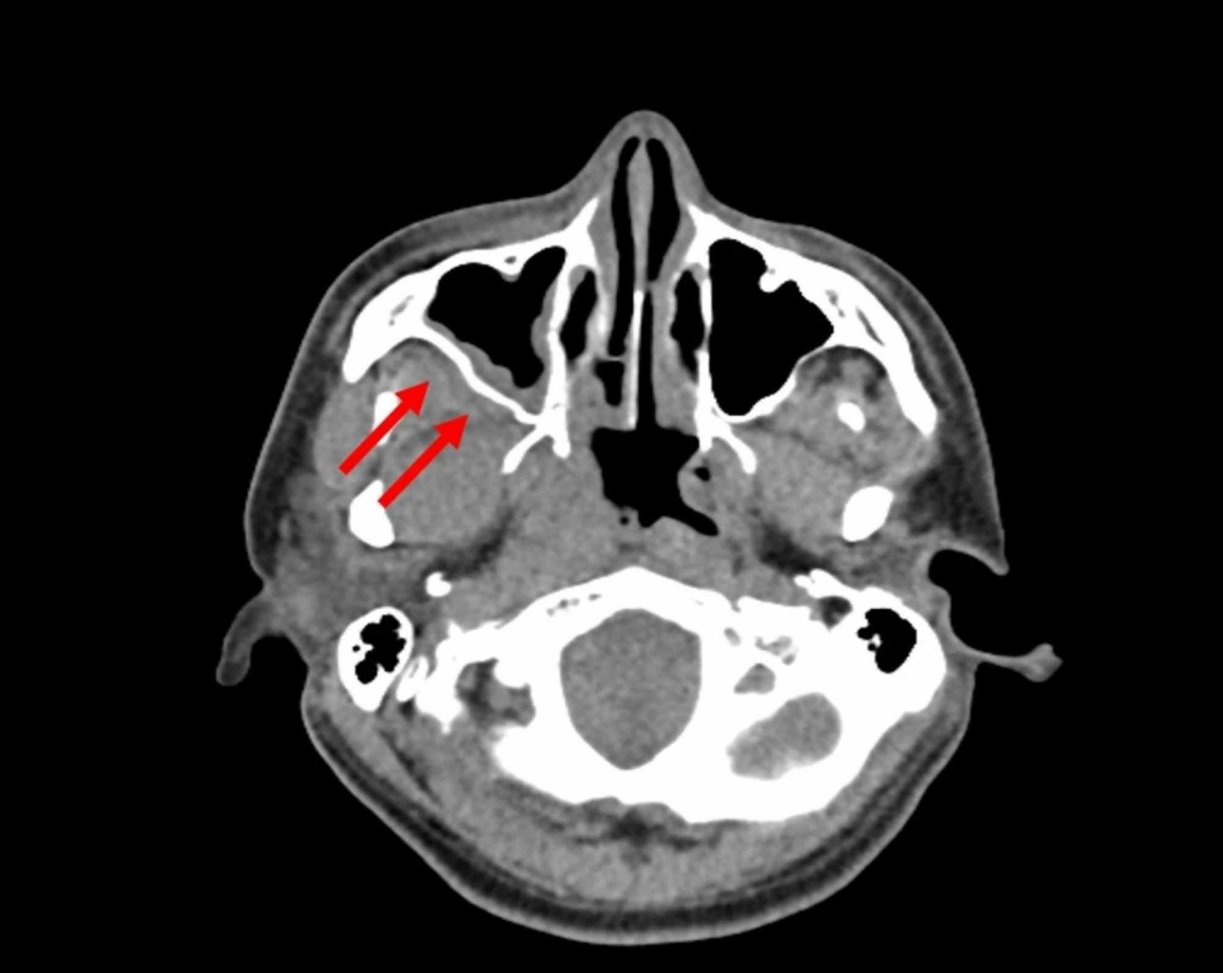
Axial non-contrast CT on admission. The red arrows show infiltration of the right retroantral fat plane indicating invasive disease extending through the posterior wall of the right maxillary sinus.
